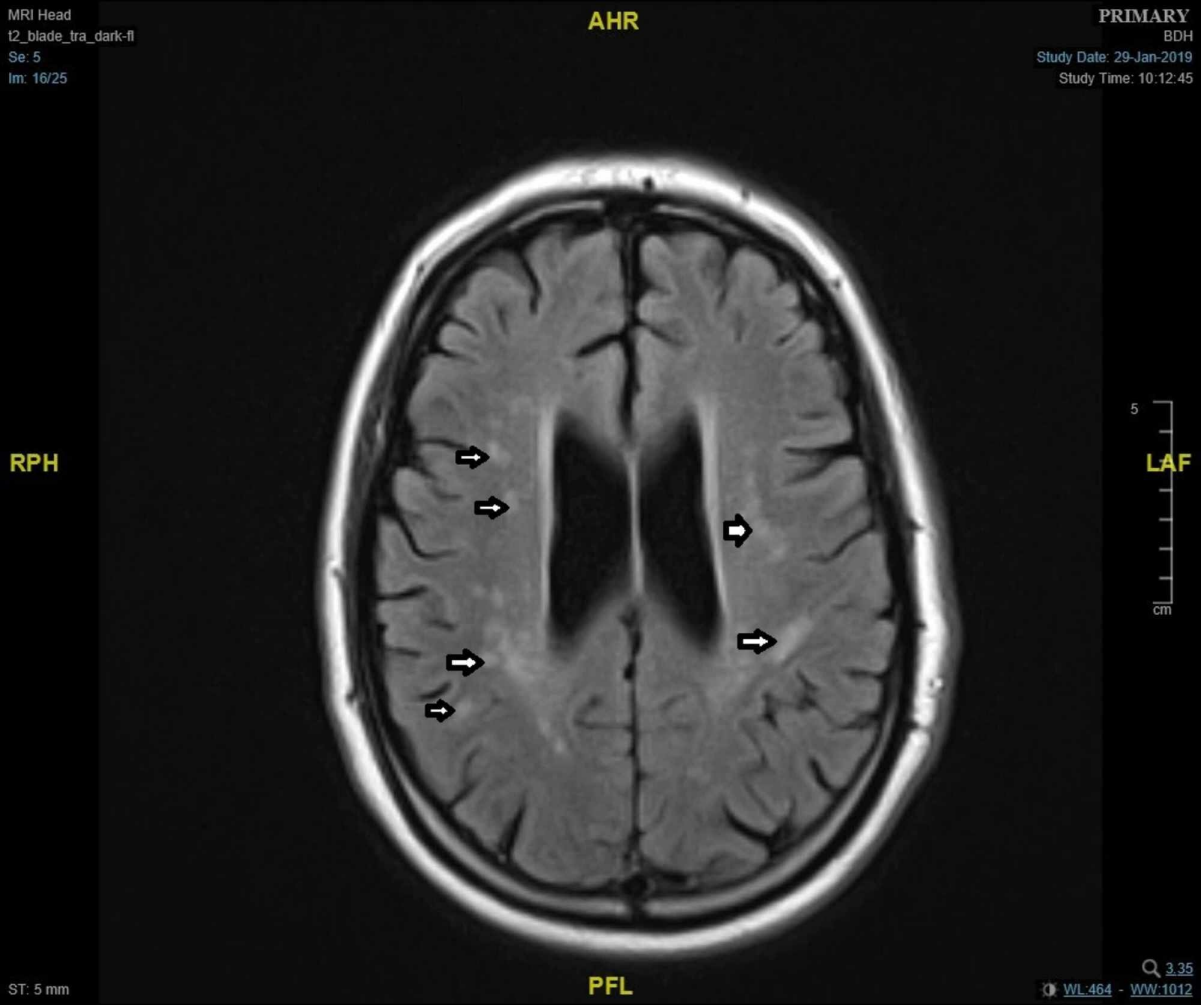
MRI demonstrating T2 hyperintense foci in cerebral white matter likely represent ischaemic gliosis secondary to small vessel disease. This raises suspicion of an acute demyelination.
